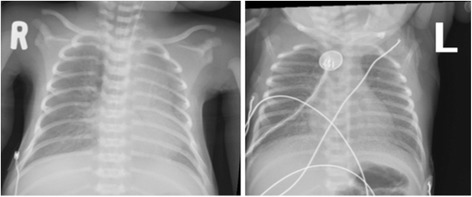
Chest radiograph of a neonate with neonatal pneumonia
